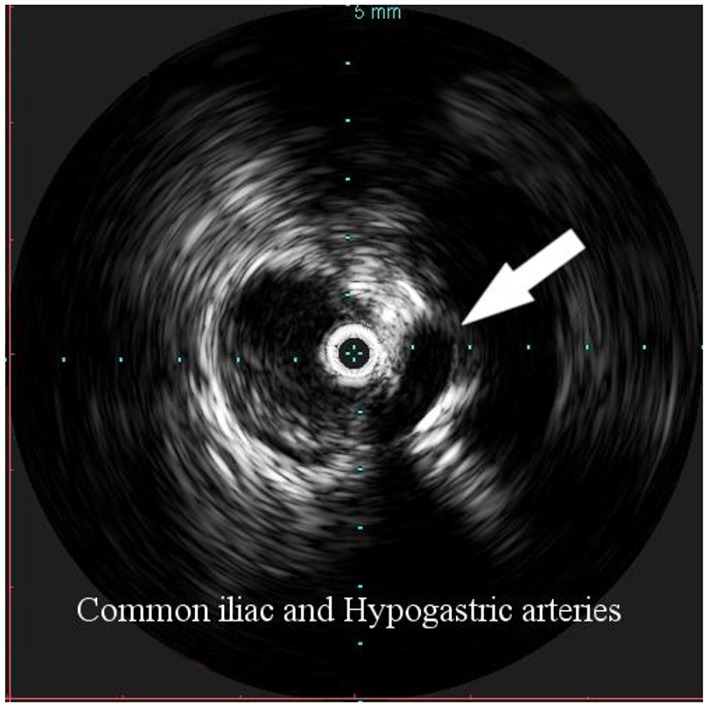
Intravascular ultrasound (IVUS) identification of the hypogastric artery offspring (arrow), in order to identify the distal landing zone of the graft.
